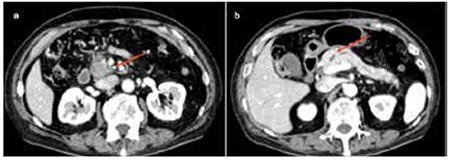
Computed tomography scans of the abdomen: (a) A 24x20-mm tumor mass was noted in the head of the pancreas (red arrow). (b) The main pancreatic duct was dilated, but there was neither vascular abnormality nor lymph node swelling (red arrow).
